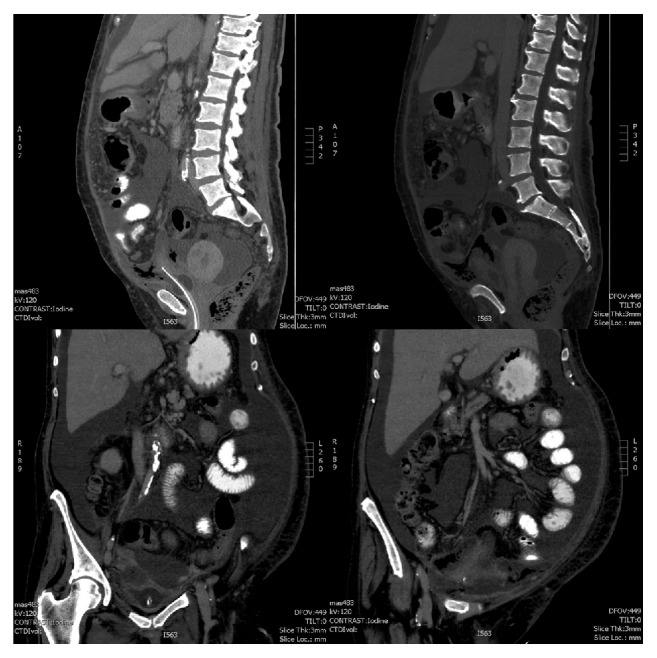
Coronal/sagittal abdominal CT scan images suggesting bladder rupture and diffuse peritonitis.
